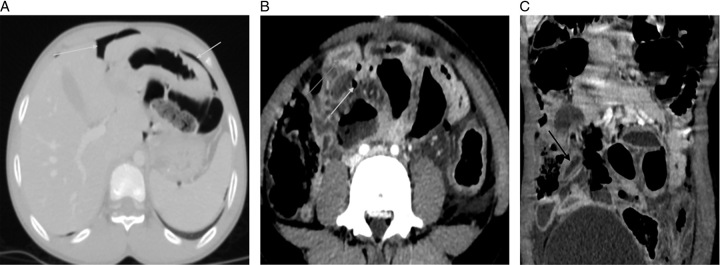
A, Lung window axial CECT image of the abdomen at the hepatic level showing free in the perigastric regions (white arrows). B, Contrast-enhanced axial image of the abdomen showing a thickened wall of the terminal ileum (gray arrow) with suspicious wall defect and air foci in terminal ileum (white arrow). C, Coronal contrast-enhanced CT image showing the thickened bowel loops in the distal ileum (black arrow).
